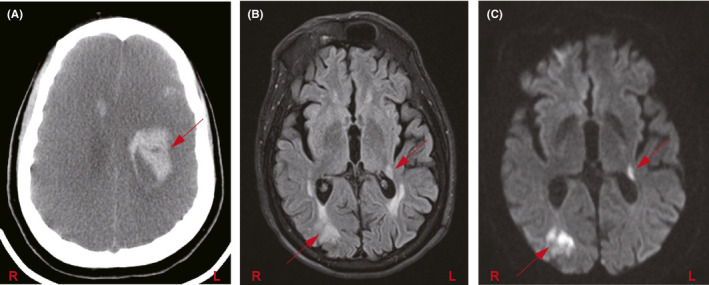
Neurological complications in COVID‐19 patients. (A) Non‐contrast CT head shows diffuse loss of gray‐white differentiation and sulcal effacement, consistent with global hypoxic ischemic encephalopathy. Additionally, there are multifocal intracranial hemorrhages, including a large hematoma in the left fronto‐parietal deep white matter (red arrow); (B) T2‐FLAIR and (C) DWI MRI brain sequences in a patient show right parieto‐occipital and left posterior thalamocapsular acute infarcts (arrows)
